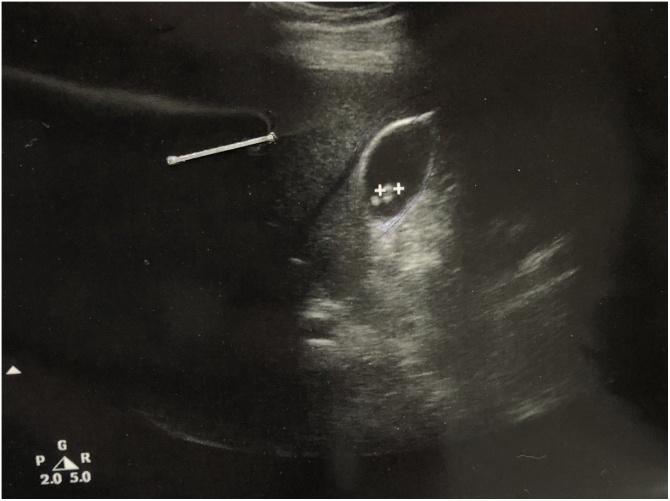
An ultrasound picture of the gall bladder showing the presence of gall stones with thick bile and slight increase in the wall thickness.
